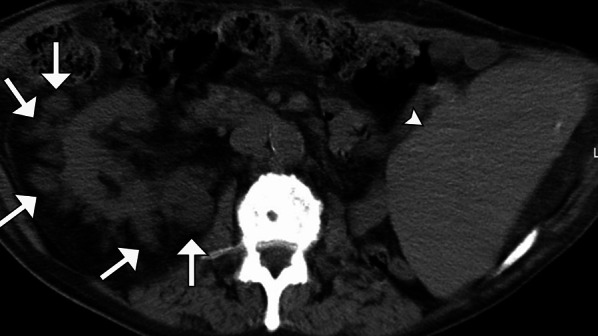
Renal extramedullary hematopoiesis.* Axial noncontrast CT in an anemic patient demonstrates splenomegaly (arrowhead) and multiple right renal masses (arrows). The constellation of findings is suspicious for underlying lymphoma. A biopsy of one of the renal masses shows hematopoietic tissue consistent with extramedullary hematopoiesis
